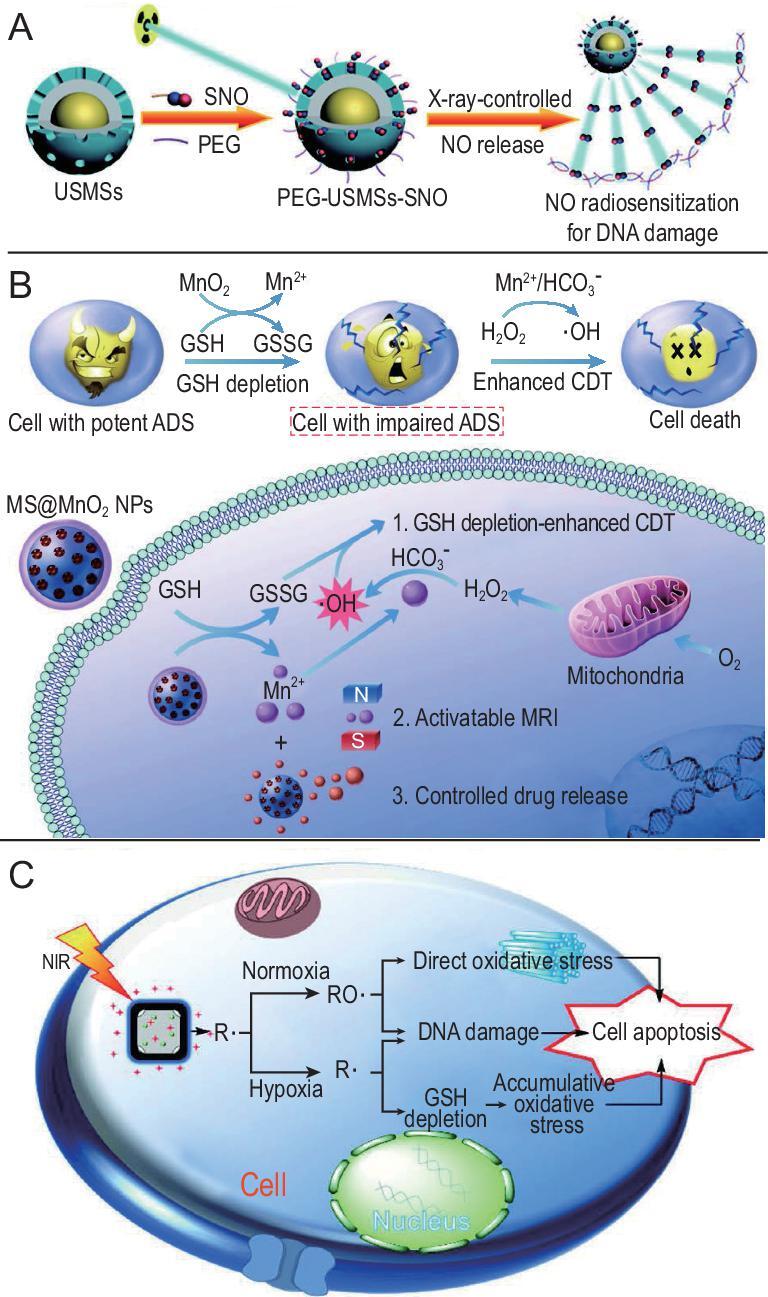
Diminishing oxygen dependence of nanomaterials for hypoxic tumor therapy. (A) X-ray controlled NO release to sensitize radiotherapy for DNA damage. Reproduced with permission from [126]. Copyright 2015, Wiley-VCH. (B) The mechanism of MnO_2_ as a smart chemodynamic agent for enhanced CDT of tumors. Reproduced with permission from [141]. Copyright 2017, Wiley-VCH. (C) Therapeutic mechanism of the free radicals under different oxygen tensions. Reproduced with permission from [146]. Copyright 2017, Wiley-VCH.
